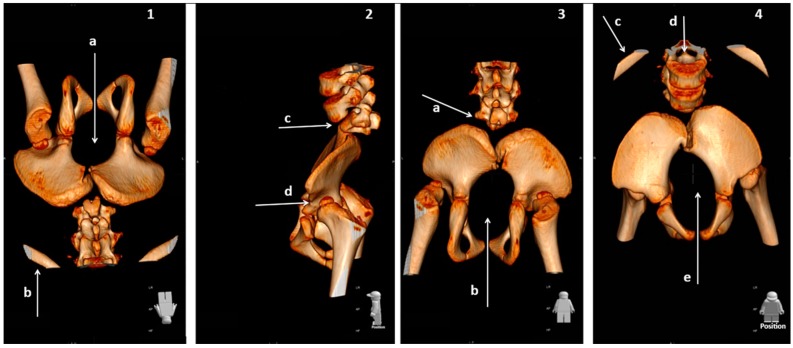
3D reconstruction of a CT-SCAN showing the vertebral and hip abnormalities. (1) Inverted position for a better view of the hypoplastic L3 vertebra. The arrow marked “a” shows the sacral agenesis, while arrow b indicates the 12th rib (to show where the lumbar column begins); (2) In this sagittal view, arrow c shows the hypoplastic L3 vertebra and arrow d shows the lack of articular congruence between the head of the femur and the abnormal left hip acetabulum; (3) Posterior view, in which arrow a shows the abnormal L3 and arrow b shows the sacral agenesis. Notice too the iliac bones articulated in the midline, the rotation of the hips, the incomplete development of both acetabulae, and the luxated femoral heads; (4) Oblique view for seeing the spinal cord (arrow d). Arrow c shows the 12th rib and arrow e shows the sacral agenesis. Note, again, the articulation of the iliac bones and the rotation of the left hip.
